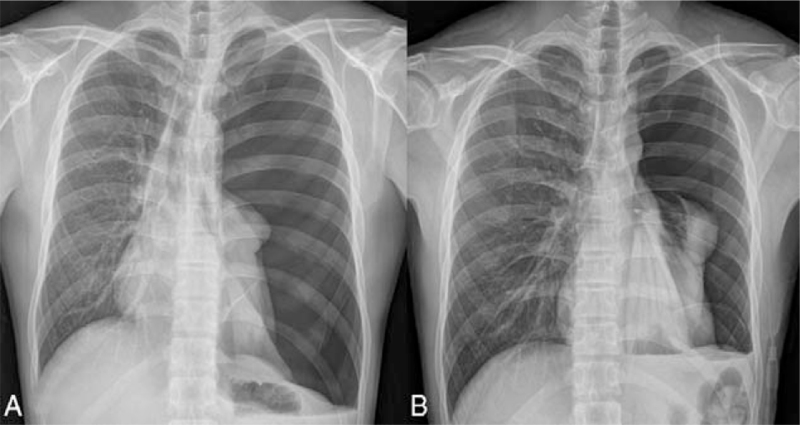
Chest x-ray at the time of admission show left sided complete pneumothorax with total lung collapse (A). A follow up chest x-ray at the day after chest tube insertion show the left lung is not expanded much (B).
